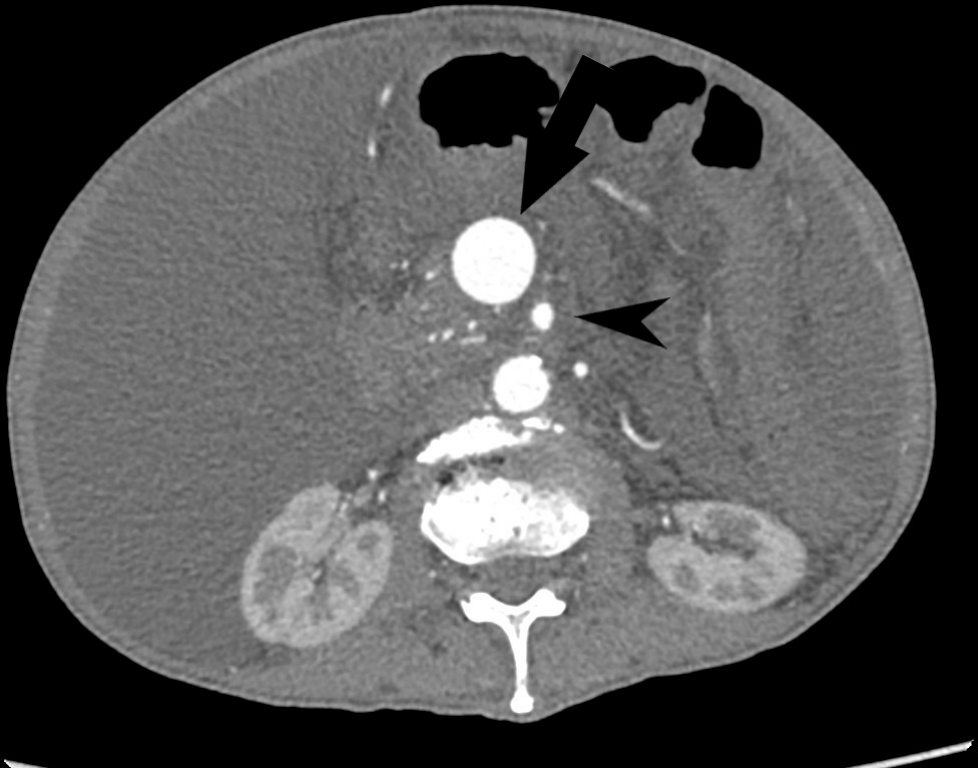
Contrast-enhanced axial CT (arterial phase) shows early enhancement of the superior mesenteric vein (arrow) which is grossly distended and also shows similar enhancement to adjacent superior mesenteric artery (arrowhead).
